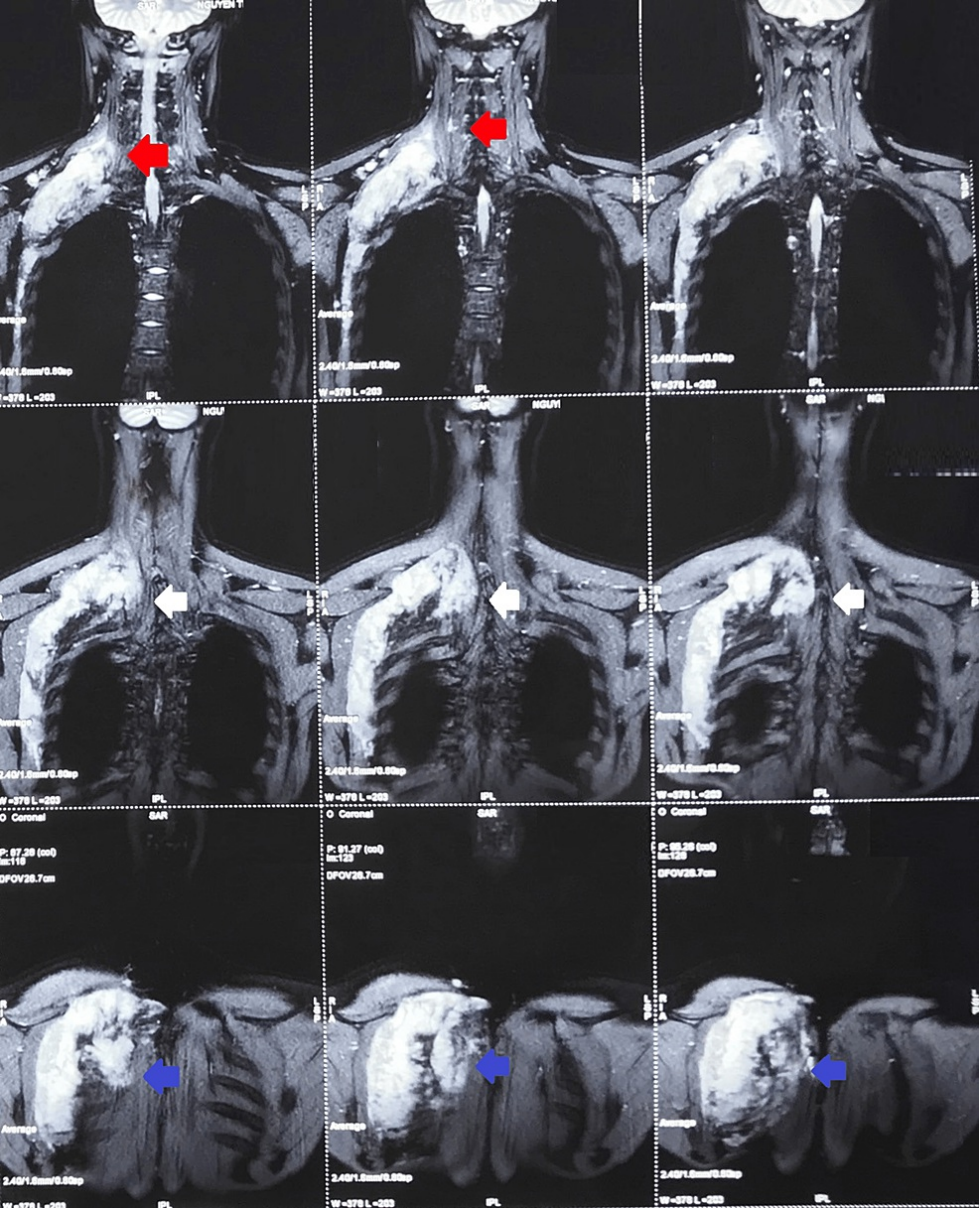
Magnetic resonance imaging (MRI) scans conducted on the upper back region There is a notable mass observed within the rhomboid muscles (write arrows), encompassing both the minor and major parts. This lesion demonstrates hyperintensity on T2-Weighted (T2W) images. In post-contrast images, the mass reveals a homogeneous strong enhancement. The lesion exhibits invasive characteristics, with evidence of infiltration into the longissimus cervicis muscle (red arrows) and the infraspinatus muscle (blue arrows). The MRI features are suggestive of a desmoid tumor in the rhomboid muscles with invasion into the longissimus cervicis and infraspinatus muscles.
